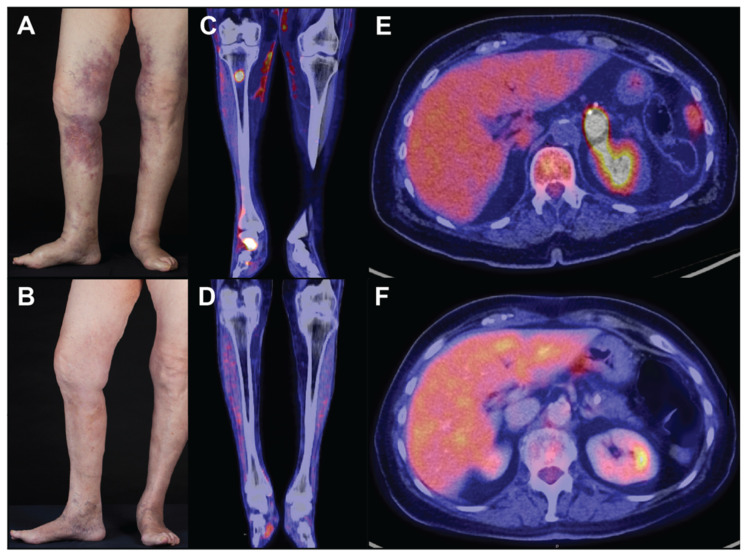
Macroscopic findings in IVLBCL: (A,B) cutaneous lesions before (A) and after (B) two cycles of R-CHOP; (C–F) PET-CT scans of cutaneous and osseous lesions of the legs before (C) and after (D) six cycles of R-CHOP. Adrenal gland lesion before (E) and after (F) six cycles of R-CHOP. PET-CT scanning showed increased uptake of fluorodeoxyglucose (^18^F-FDG) in the subcutaneous tissue of both legs, the right tibia, various osseous structures of the right foot (maximal standardised uptake value (SUVmax) = 3.0–21.5), and a nodular lesion in the left adrenal gland (SUVmax = 31).
